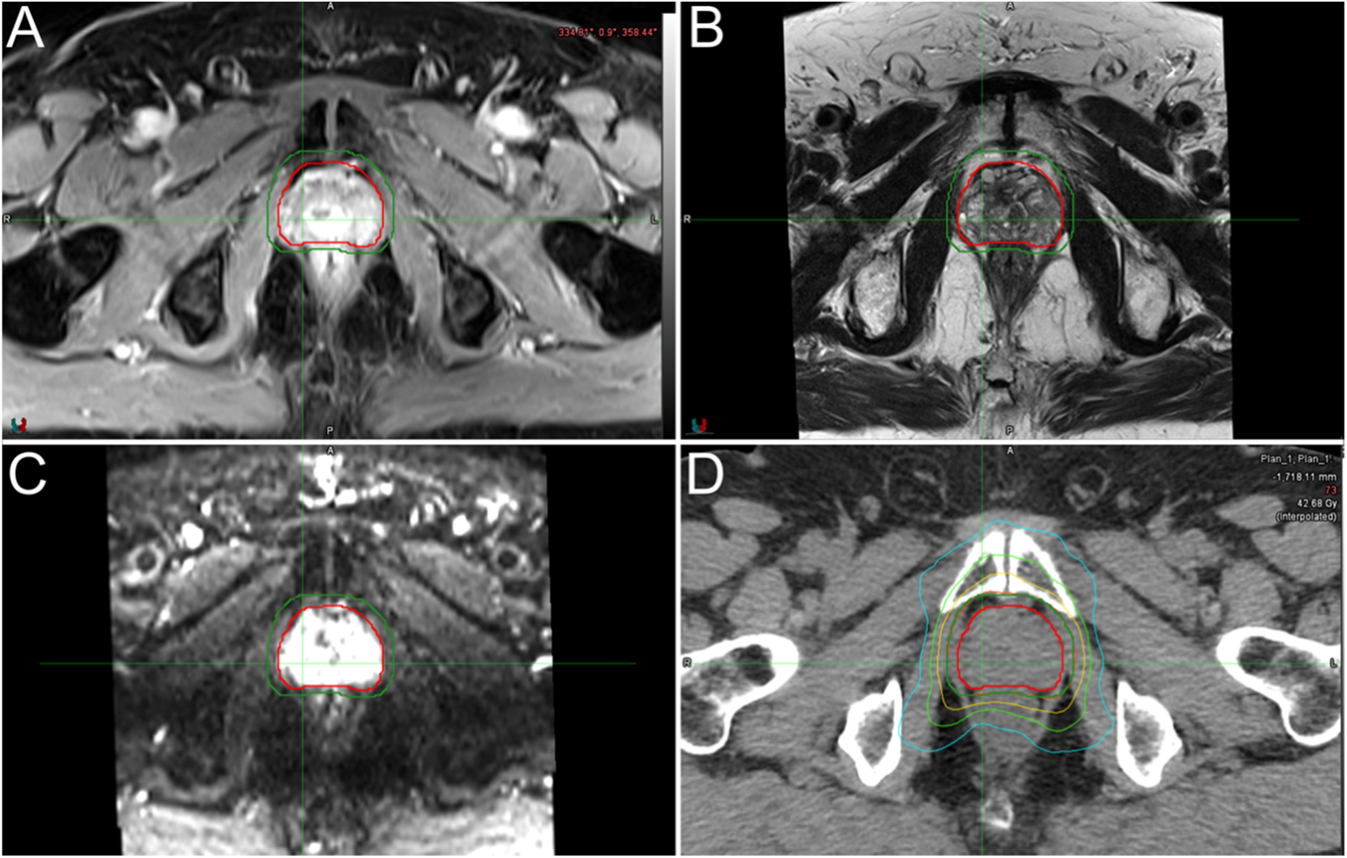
Contrast-enhanced fat-saturated T1w (A), T2w (B) images, b1000 DWI (C), and related centering CT (D) with superimposed GTV (red line), PTV (green line) and distribution of doses (D) in a patient with prostate cancer
